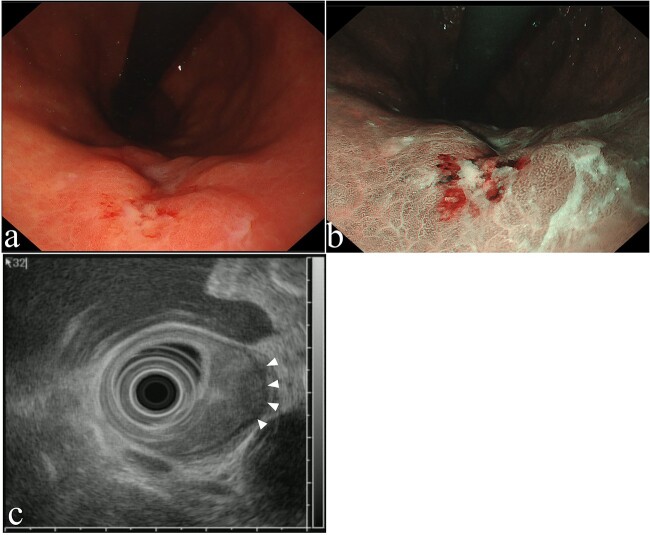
Upper gastrointestinal endoscopy findings. (a) 15-mm 0-IIc lesion, (b) narrow-band imaging. (c) Endoscopic ultrasound image revealed a low-echoic lesion with invasion of the muscularis propria layer (arrowheads).
